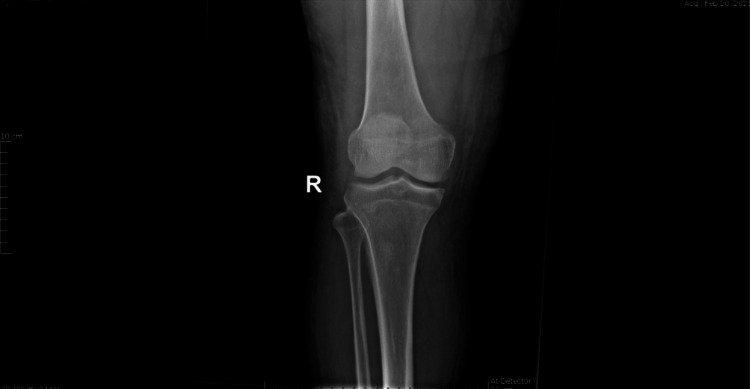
AP X-ray of the right knee showed no osteolytic changes PA: posteroanterior
